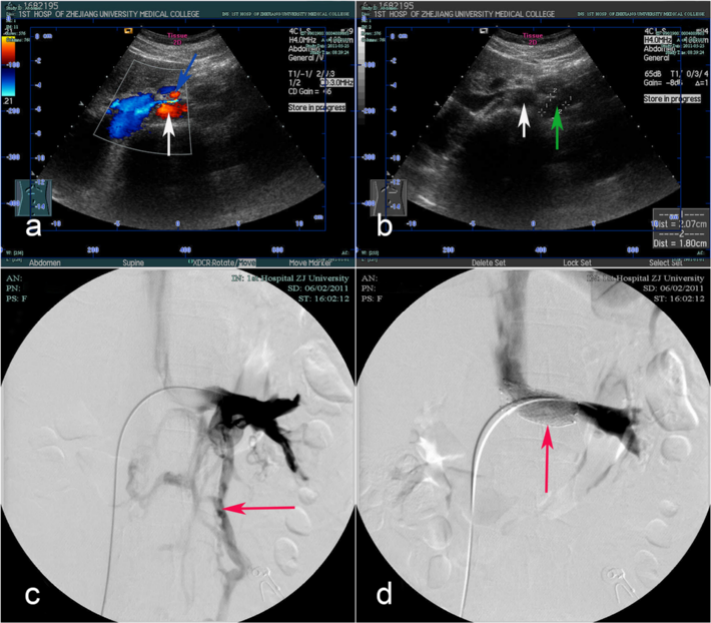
The images of the duplex ultrasound and the left renal venography. a, Right transverse image: Duplex ultrasound demonstrated the compressed left renal vein between the aorta (white arrow) and the superior mesenteric artery (blue arrow), and the left renal vein was pressed like a beak. b, Left transverse image: Duplex ultrasound demonstrated a narrowing of the left renal vein at the aortomesenteric portion and varices of left gonadal vein (green arrow) arising from the left renal vein on the left of aorta (white arrow). c, Before extravascular stenting, left renal venography demonstrated there was obstruction of left renal venous outflow and perihilar varices (red arrow). d, After endovascular stenting (red arrow), left renal venography showed unobstructed blood outflow and full stent expansion without obvious protrusion of the stent in the inferior vena cava
